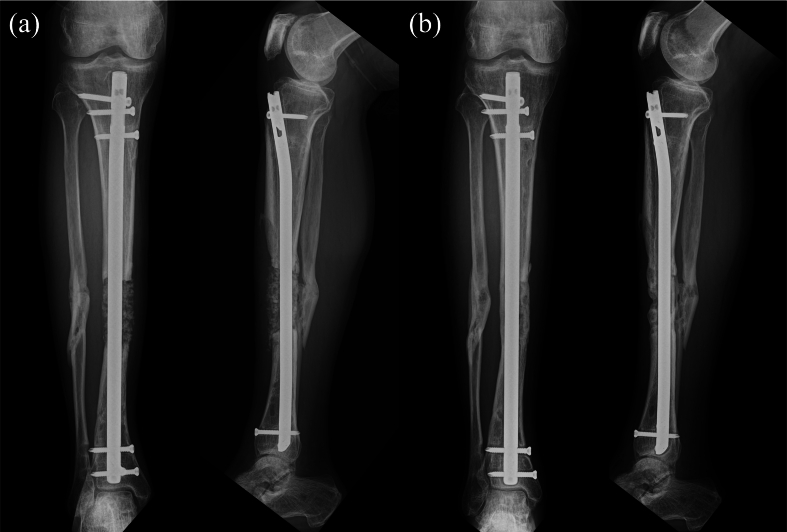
Radiographic images (a) immediately following cancellous bone and beta-tricalcium phosphate grafting and (b) at 10 months after.
